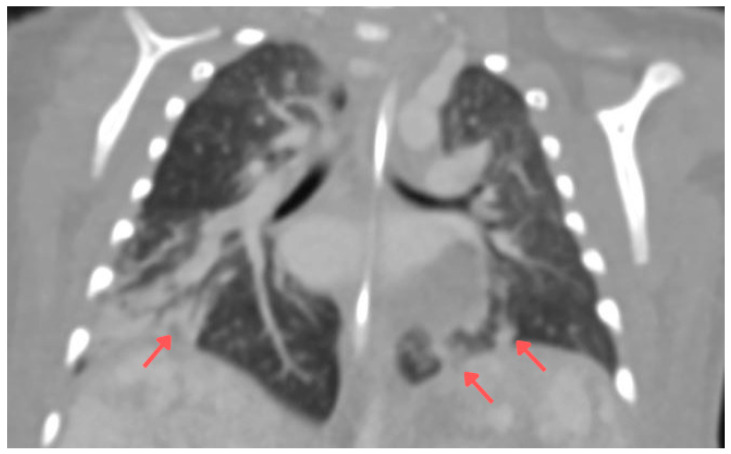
Contrast-enhanced chest CT (coronal section) performed during the first week of hospitalization. Some lesions were marked with arrows.
